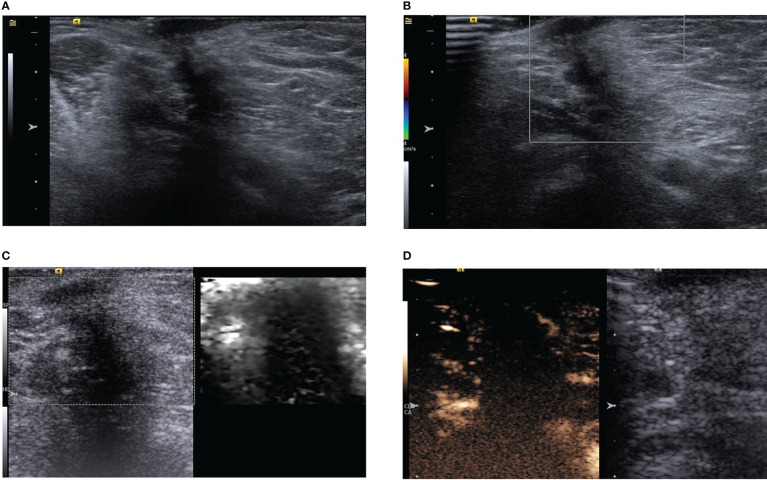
(A) Ultrasound showing a hypoechoic subcutaneous mass in the left axilla with an aspect ratio greater than one and acoustic shadowing. (B) Color Doppler shows no obvious blood supply in the mass. (C) Acoustic radiation force impulse model. (D) In the arterial phase, the tumor is slightly enhanced in the periphery, but not in the interior.
